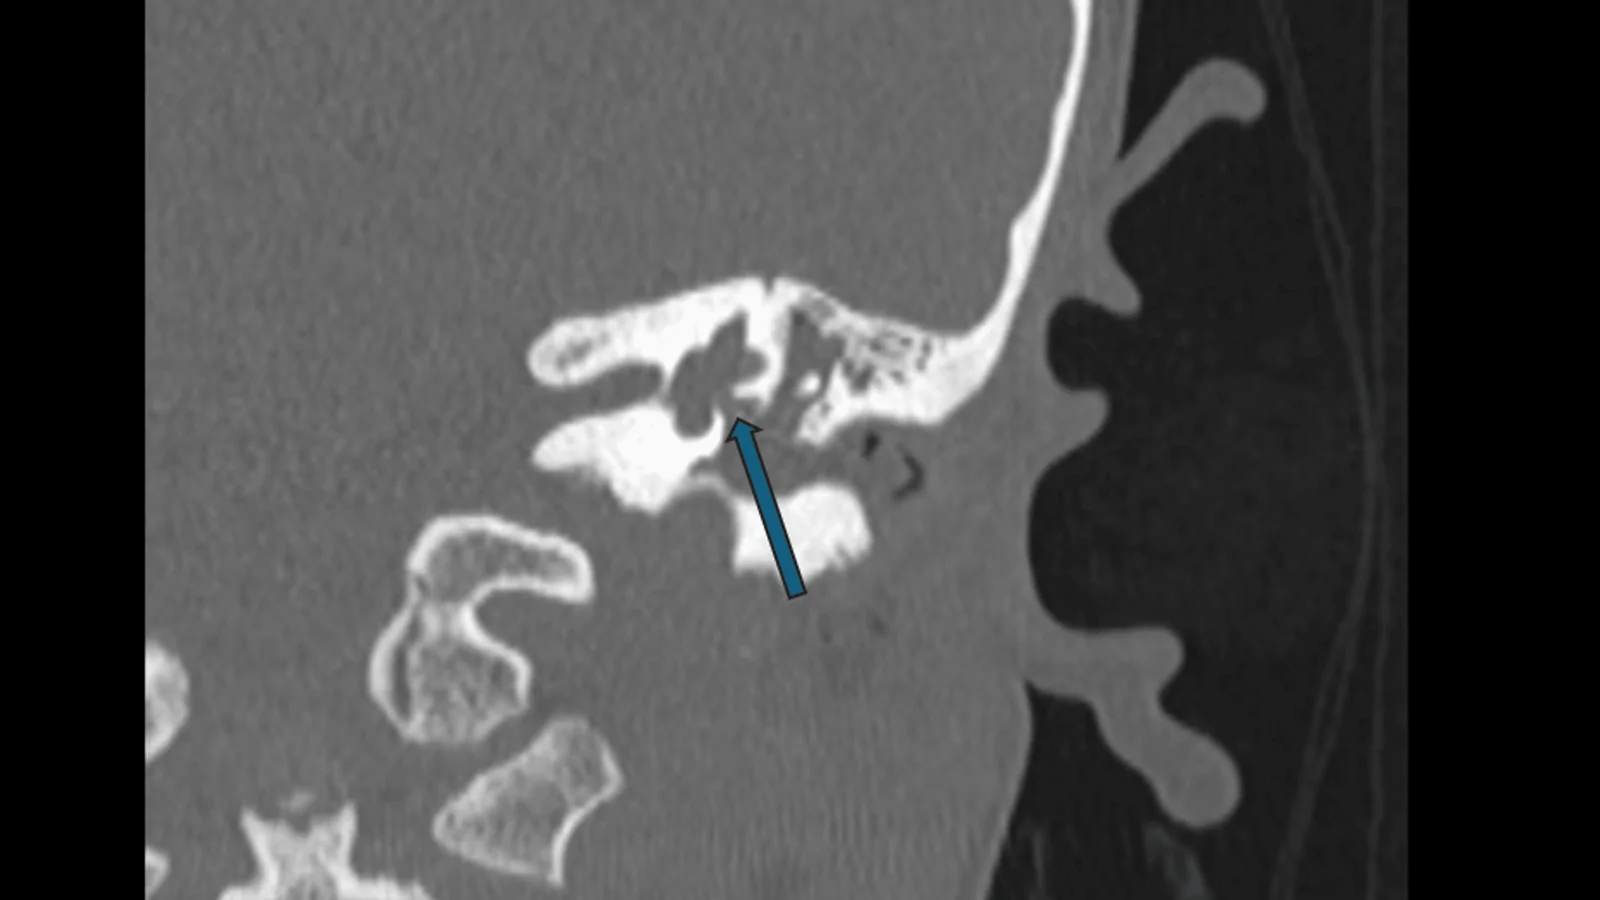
Coronal CT of the left temporal bone demonstrating a cystic cochleovestibular malformation (common cavity deformity) with a focal bony deficit connecting the cystic cavity to the middle ear space (blue arrow). This abnormal communication corresponds to the pathway through which CSF was found to egress intraoperatively from the vestibule through the oval window.
